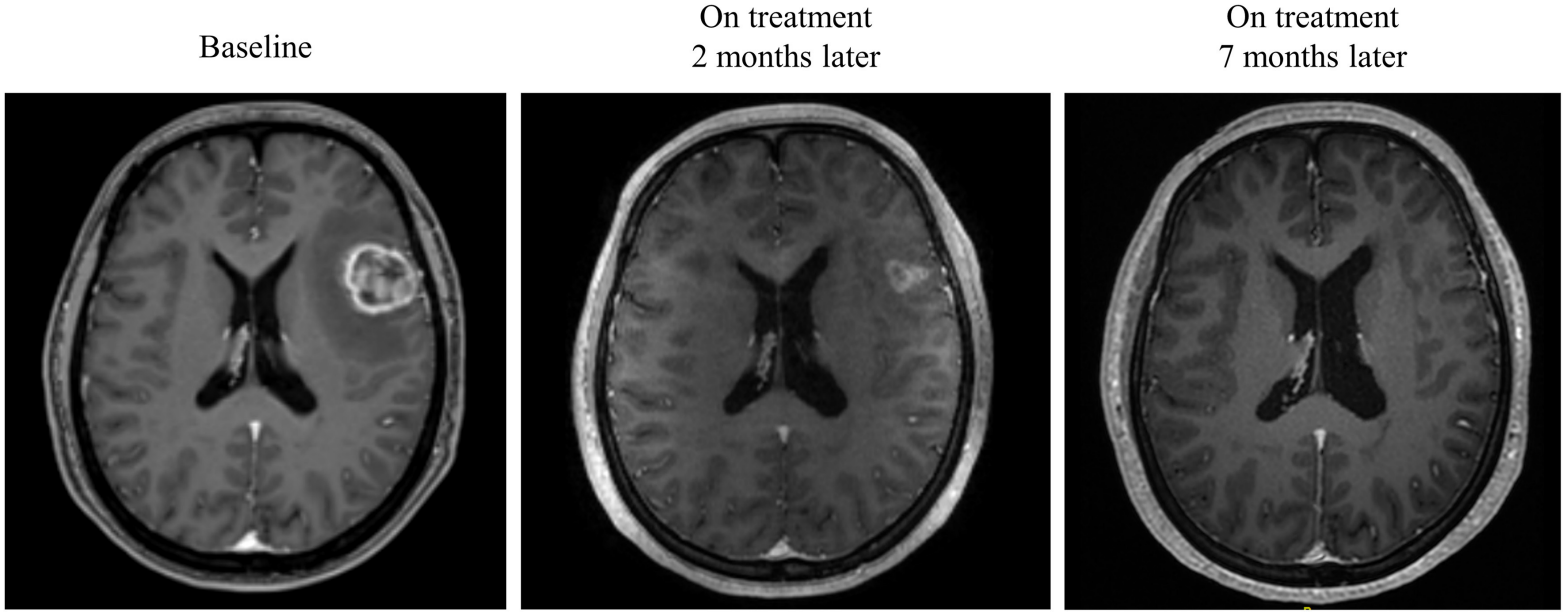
Radiological examples of intracranial complete response. A 40-year-old female diagnosed with melanoma BM was given anti-PD-1 therapy and radiotherapy. MRI images show a complete response 7 months later.
